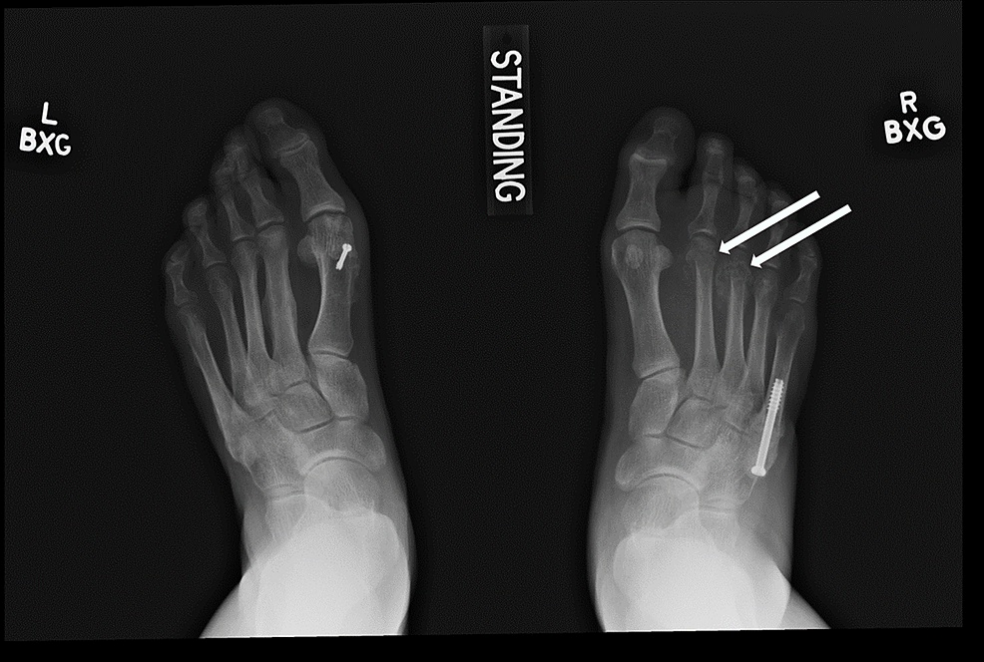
Anteroposterior radiograph of bilateral feet demonstrating second and third metatarsal neck fractures of the right foot (arrows).
